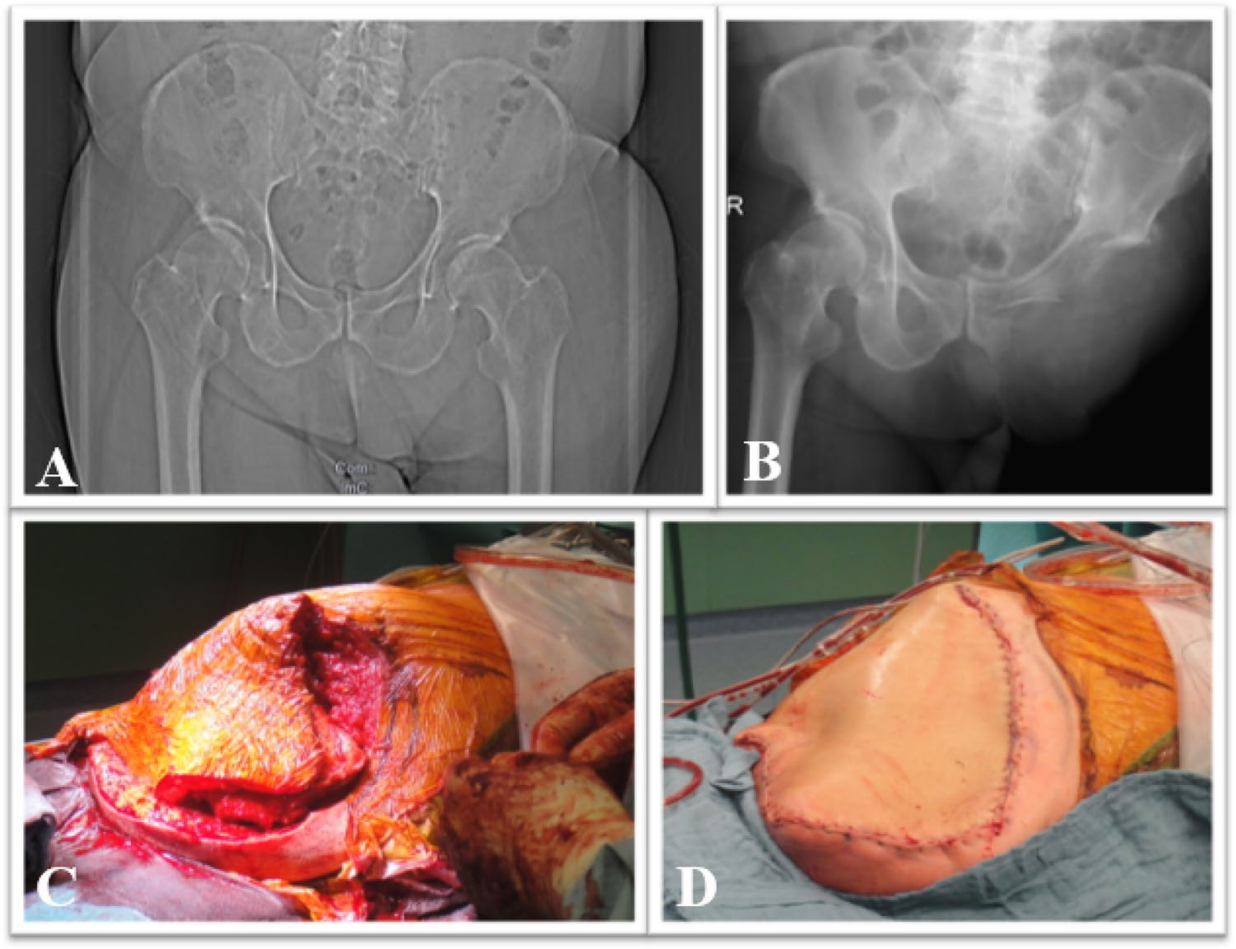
72 year-old male patient with recurrence of unclassified soft tissue sarcoma and performed external hemipelvectomy. A Preoperative X-ray of the pelvis (a.p.). B Postoperative X-ray of the pelvis(a.p.). C Intraoperative image of the external hemipelvectomy before and D after wound closure
